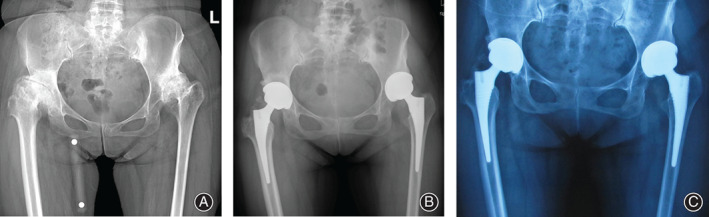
Preoperative (A) anterior–posterior X‐ray highlighted a bilateral DDH (right hip as Crowe II and left hip as Crowe III) in a 47‐year‐old female patient (Crowe index: 0.13 in right hip and 0.16 in left hip). The postoperative (B) anteroposterior radiographic measurement showed that the height of rotation center was 29.7 mm in right hip and 38.5 mm in left hip. At the final follow‐up, the radiographic evaluation (C) after 8.8 years showed no osteolysis and radiolucent line.
